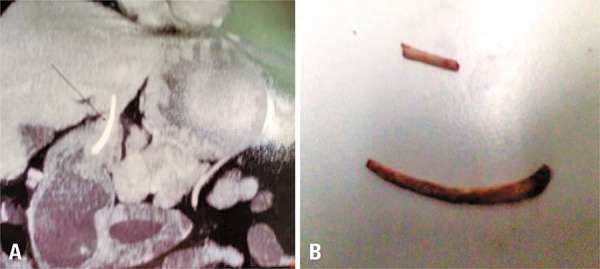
(A) Abdominal computed tomography showing the lesion involving the stomach and liver. (B) The ingested foreign body: a chicken bone
